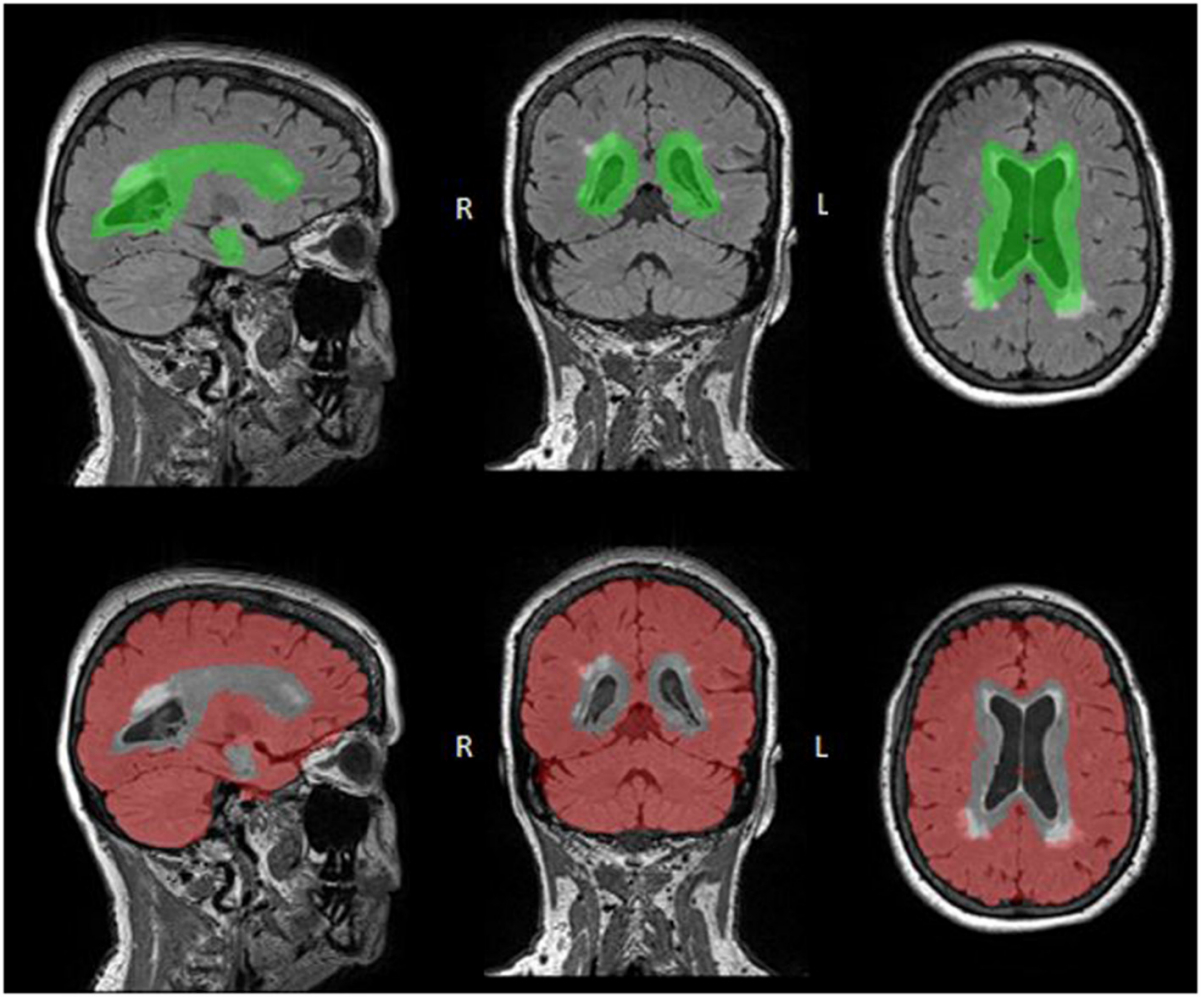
WMH Regional ROI Masks. PV (top: green) and deep (bottom: red) ROI masks are overlaid onto FLAIR images. These ROIs were defined by registering the ALVIN mask to each participant's native FLAIR space, with the area within the ALVIN mask defined as the PV ROI and the area outside defined as the deep ROI. Although both ROIs include all tissues, not just WMHs, these ROIs were multiplied by the participants WMH mask resulting in only WMH volume in each region.
